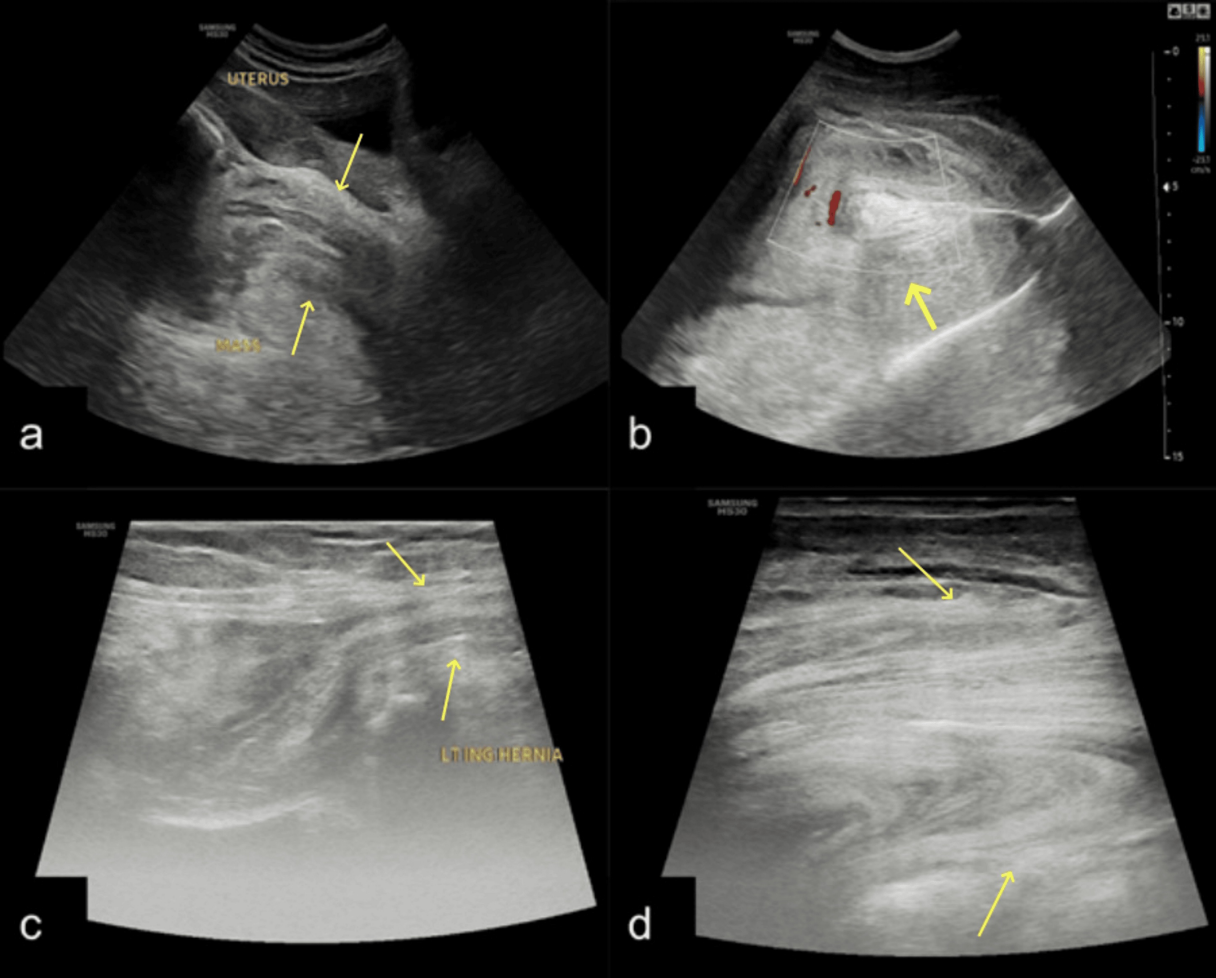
Grayscale ultrasound images obtained using curvilinear (a, b) and linear (c, d) probes show a large, solid, echogenic globular mass in the left inguinolabial region (arrows). The mass demonstrates a characteristic laminated pattern (b, d) and internal vascularity (b). Omentum-like tissue is seen extending into the left inguinal canal (arrows) (c).
